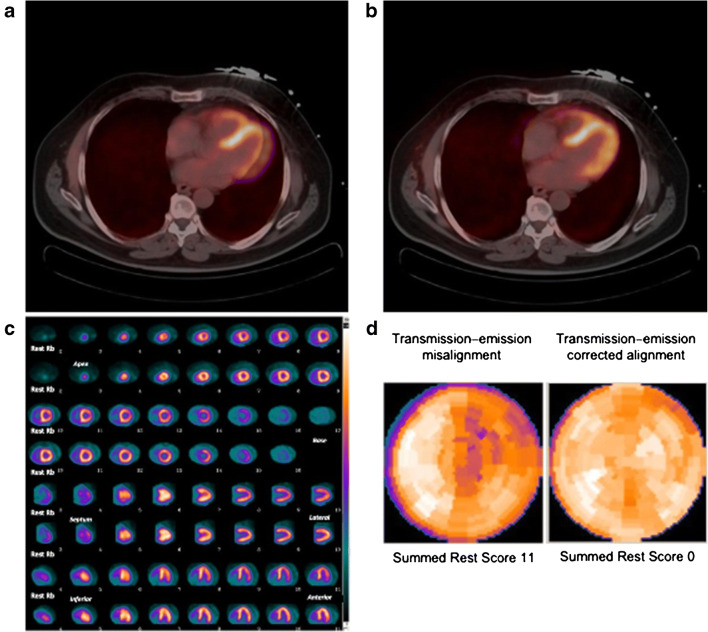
Transmission–emission misalignment example. Misalignment between CT transmission and rest ^82^Rb perfusion PET images (a) with correction of transmission–emission misalignment (b). Anterolateral perfusion defect on rest ^82^Rb perfusion images (c, upper rows) deriving from applying the incorrect attenuation coefficients during tomographic reconstruction to an area of LV myocardium overlying lung field on CT transmission scan, and normal rest perfusion study (c, lower rows) after correction, with relative polar maps (d)
